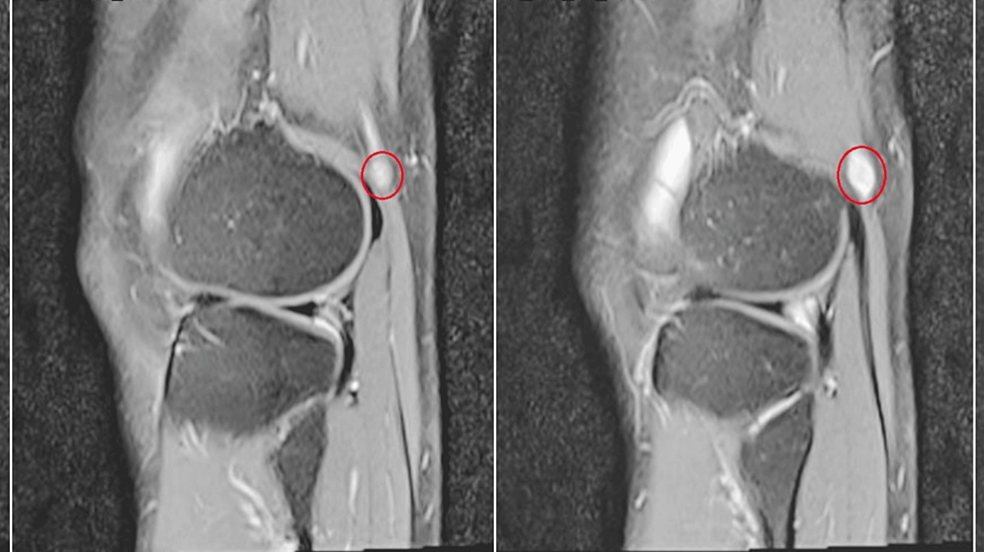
MRI showing an oval-shaped T1 Hypo/T2 hyperintense lesion(marked) in the posterolateral aspect of the left knee
